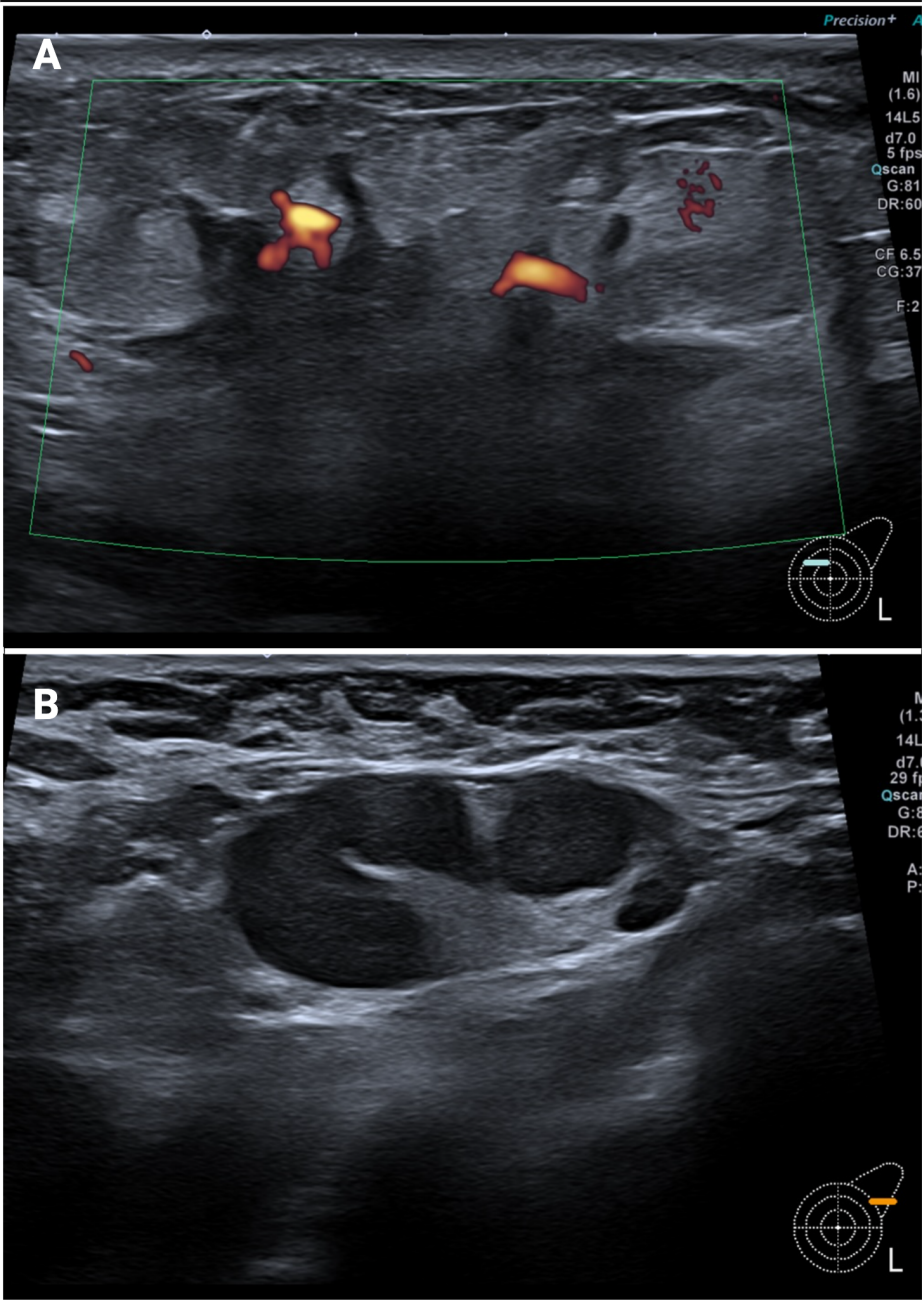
(A) Radiology assessments obtained at diagnosis. Breast ultrasound: spiculated mass highly vascularized (Doppler color positive). (B) Radiology assessments obtained at diagnosis. Breast ultrasound: enlarged axillary lymph node.
